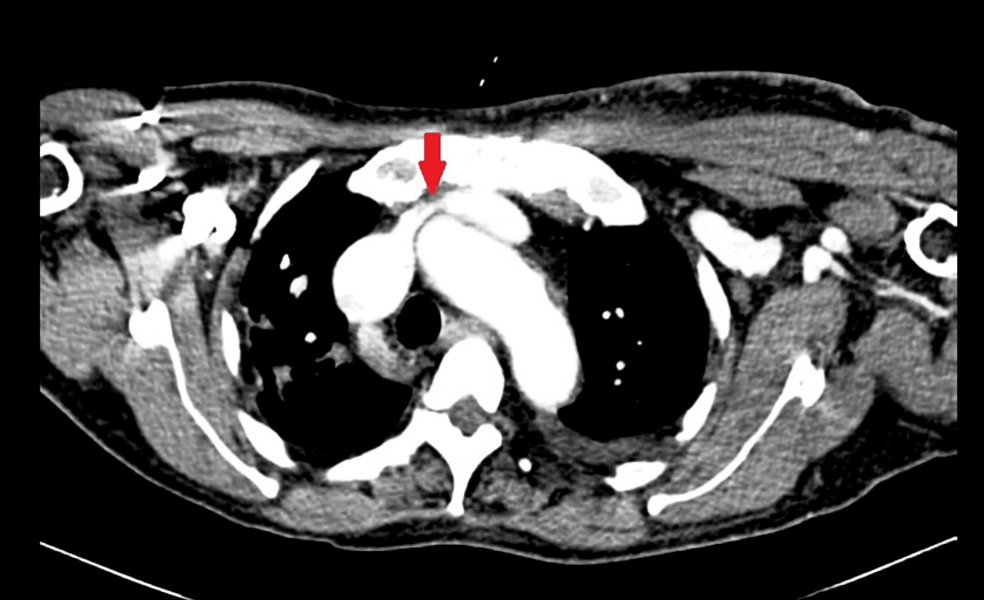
CT venogram showing narrowing of the left brachiocephalic vein (red arrow)
